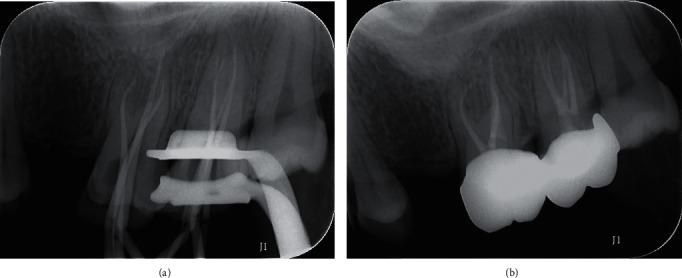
(a) Radiograph to confirm the working length, (b) postoperative radiograph.
